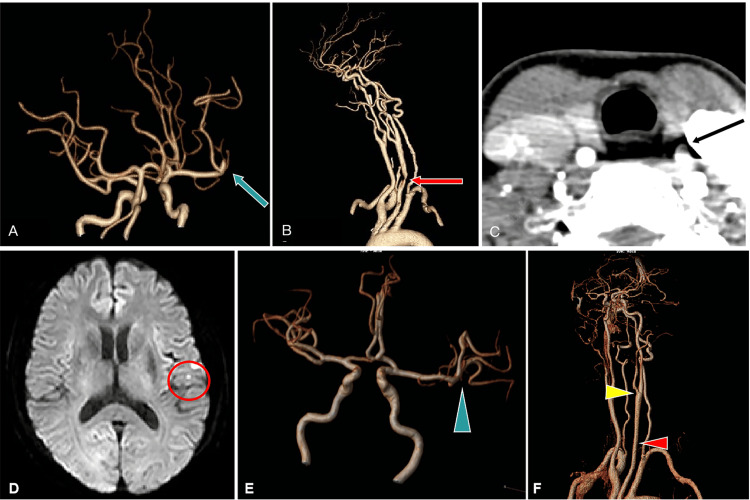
CT and MRI findings. Pre-thrombolysis reconstructed CT angiography (CTA) demonstrating occlusion of the left M2 segment of the middle cerebral artery (MCA) (A, blue arrow) and stenosis of the common carotid artery (CCA) (B, red arrow). Pre-thrombolysis CTA showing a thrombus-like shadow of the common carotid artery (CCA) (C, black arrow). Post-thrombolysis diffusion-weighted MRI revealing a small ischemic lesion in the front lobe (D, red circle). Post-thrombolysis magnetic resonance angiography (MRA) showing the occlusion of the left MCA (E, blue arrowhead). Post-thrombolysis MRA showing the stenosis of left CCA (red arrowhead) was successfully recanalized, and a filling defect can be observed in the left external carotid artery (F, yellow arrowhead).
